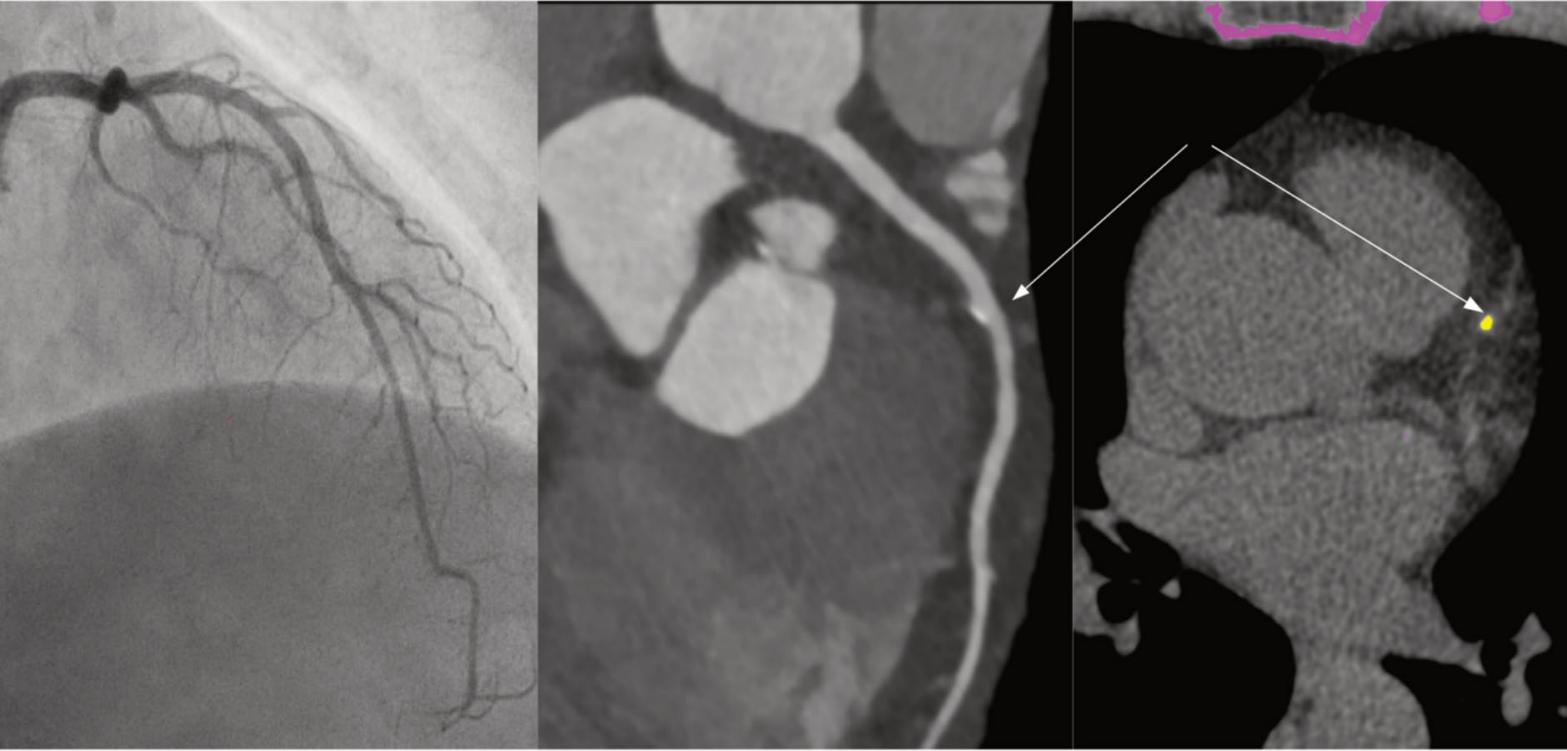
The left descending artery, with a calcified plaque in segment 6 evident only on CCTA (arrows). *CCTA* coronary computed tomography angiography
